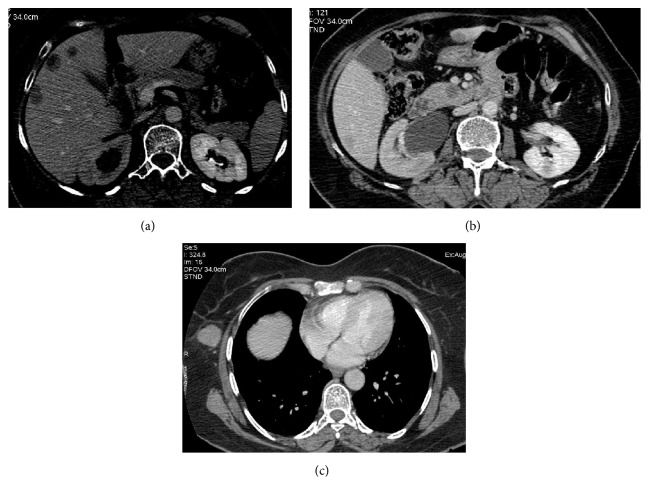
Prechemotherapy CT scan showing multiple ill-defined hypodense lesions in liver (a) and hypodense lesion in the uncinate process of pancreas (b) and in the right chest wall (c). Moderate hydronephrosis on right side secondary to lower ureteric involvement by the cervical mass (b).
